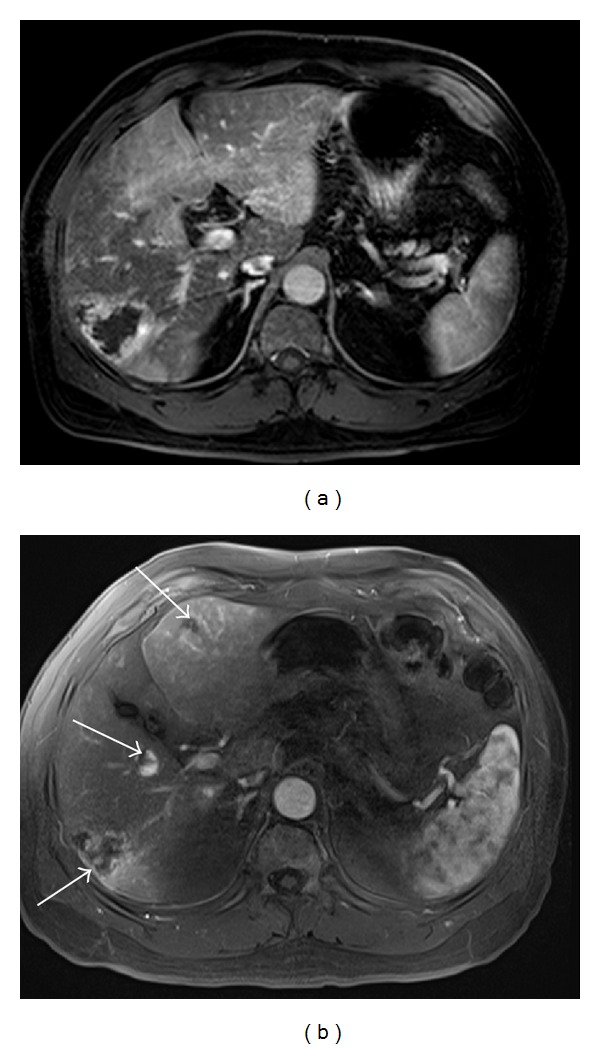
MR images obtained in a 61-year-old man with multiple hemangiomas. (a) A hepatic arterial dominant phase image using conventional 3D GRE during a 20-second breath-hold shows respiratory motion artifacts. (b) A hepatic arterial dominant phase image using the CAIPIRINHA 3D GRE sequence during a 12-second breath-hold demonstrates good image quality without artifacts, high spatial resolution, and a well-timed late arterial image and, thus, resulting in detecting an increasing number of hemangiomas (arrows).
